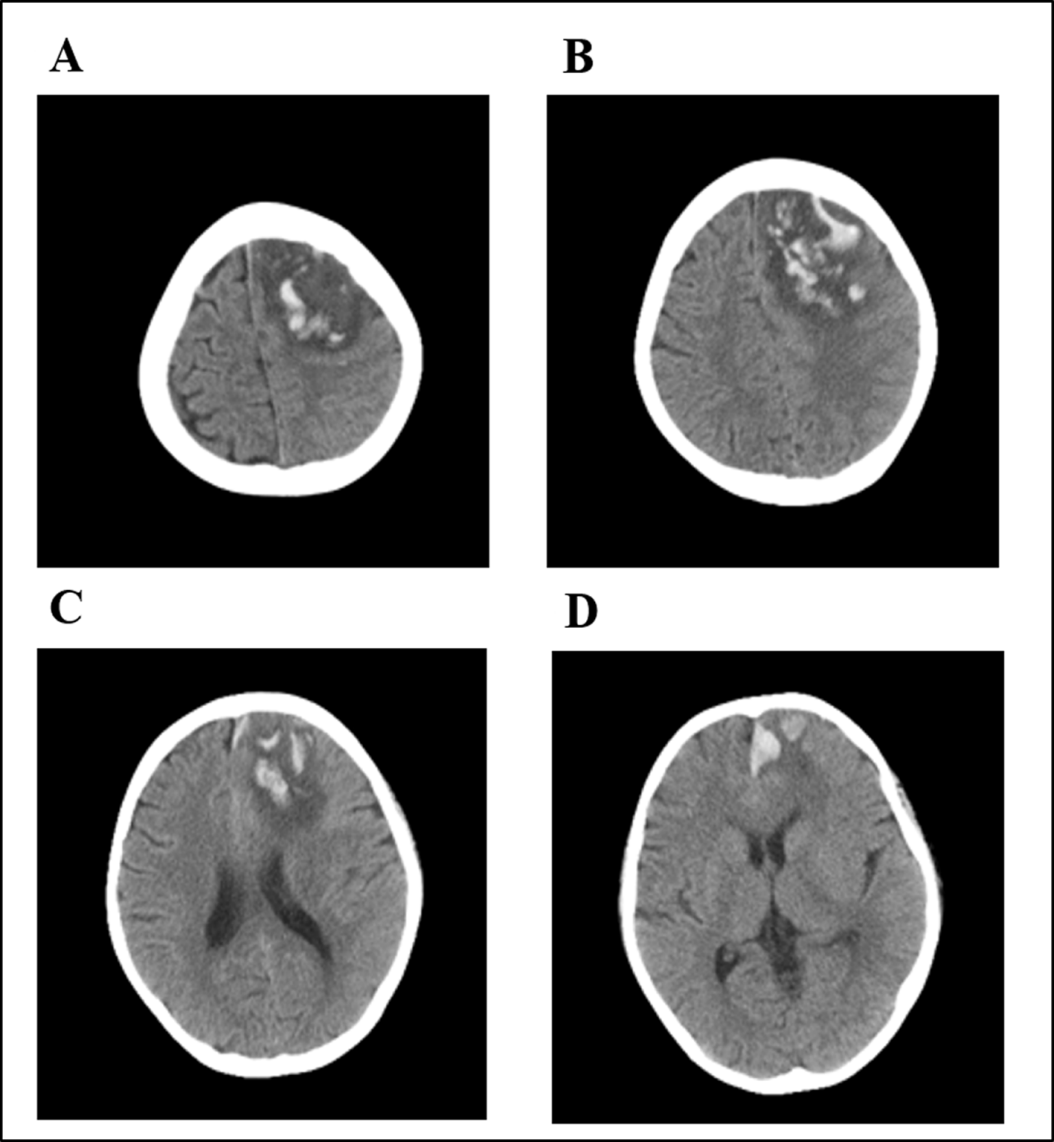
Brain computed tomography (CT) of the patient after oral low-dose MTX. Multiple hematomas were shown involving the left frontal (A), parietal (B), and temporal lobes (C) and the centrum semiovale (D).
